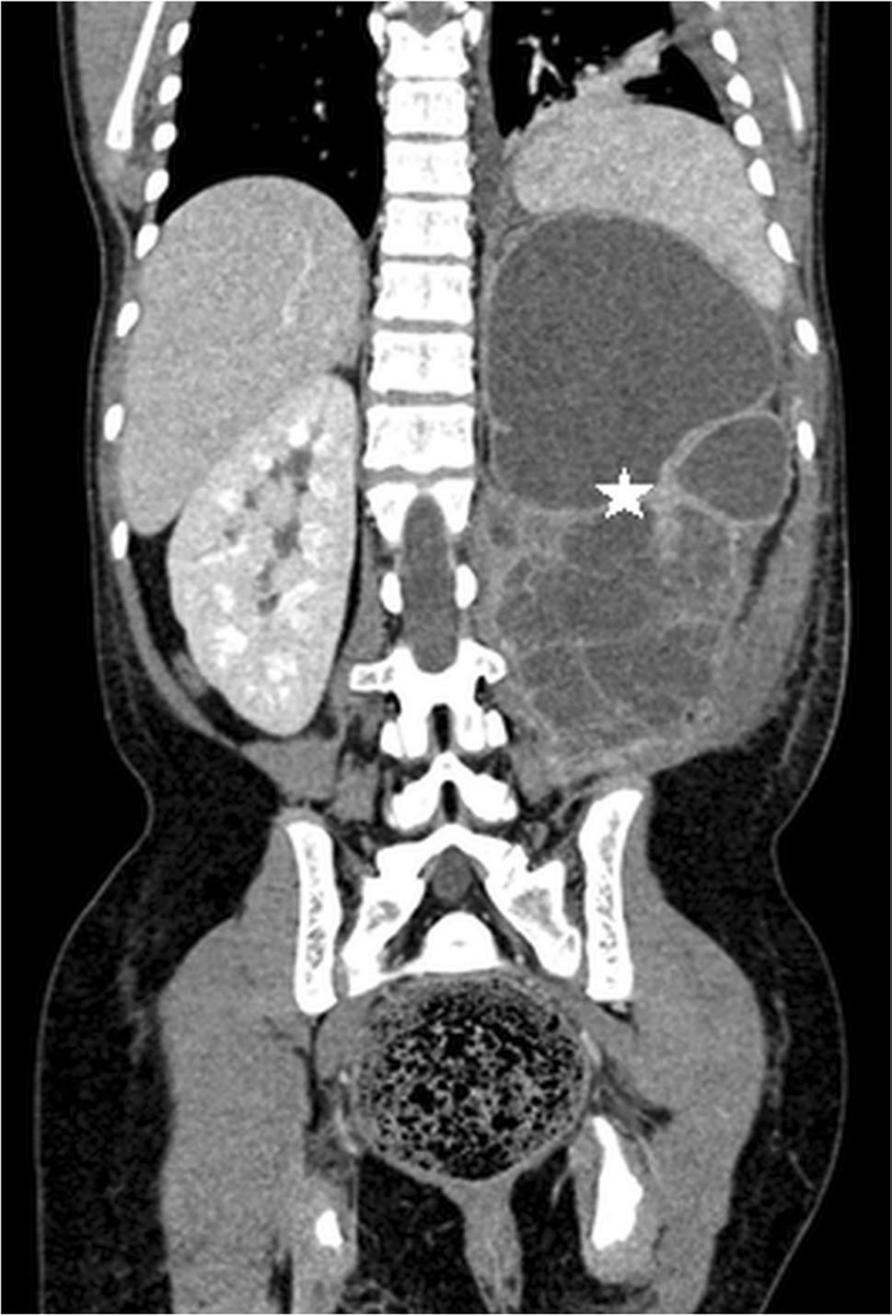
Pyonephrosis in a 13-year-old boy. Coronal contrast-enhanced CT shows a large multicystic lesion with destruction of the left kidney parenchyma (*star*) corresponding to left-side pyonephrosis as a complication of severe obstruction of the pelvicalyceal system
